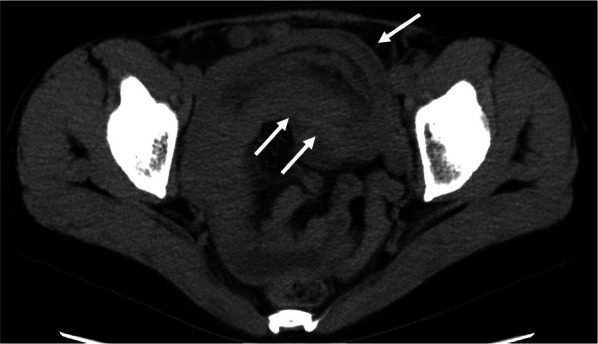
Computed tomography findings on the second admission. Computed tomography revealed the recurrence of jejunal intussusception (white arrows) without any free air or fluid
